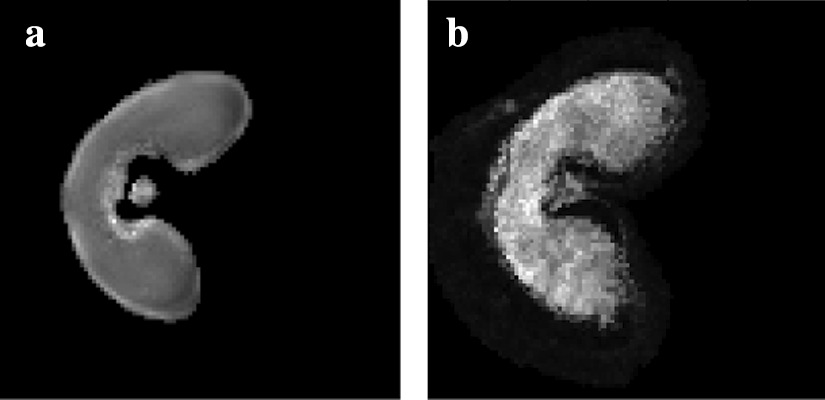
Projection of the MRI image in the NMF reduced space. Difference between a the original MRI image and the b MRI image projected in the NMF space and obtained by linear combination of the component images. Both images have similar intensities. The NMF does not result in major information loss
